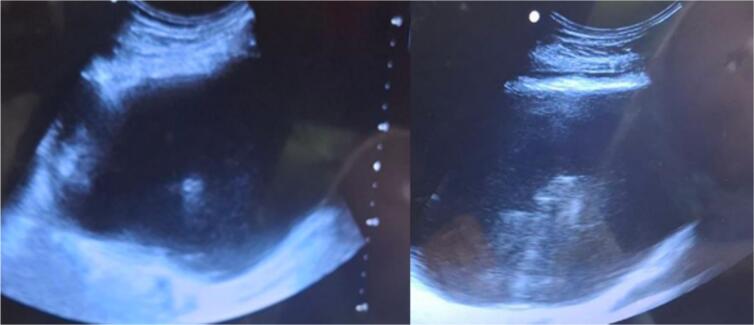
Abdominal ultrasound demonstrated evidence of bilateral psoas abscesses.
